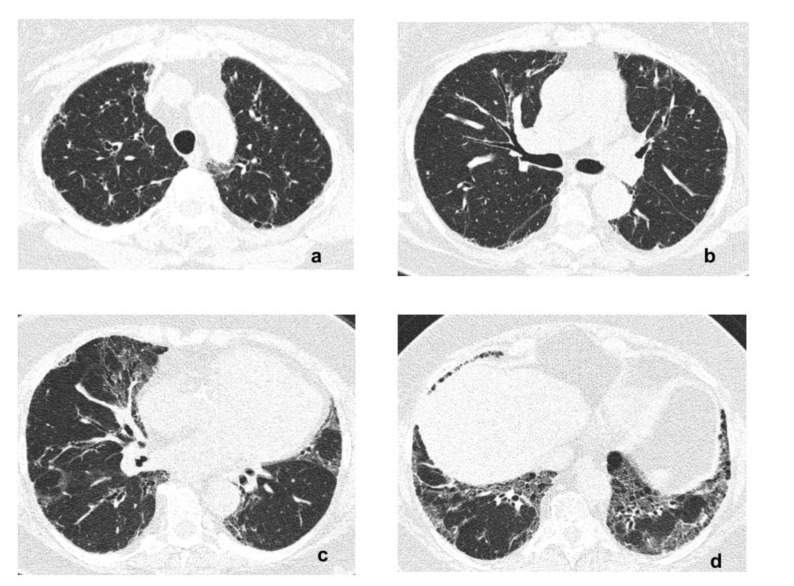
(a–d) Follow-up HRCT images (2012) show regression of ground-glass opacities and persisting reticular opacities with traction bronchiectasis.
